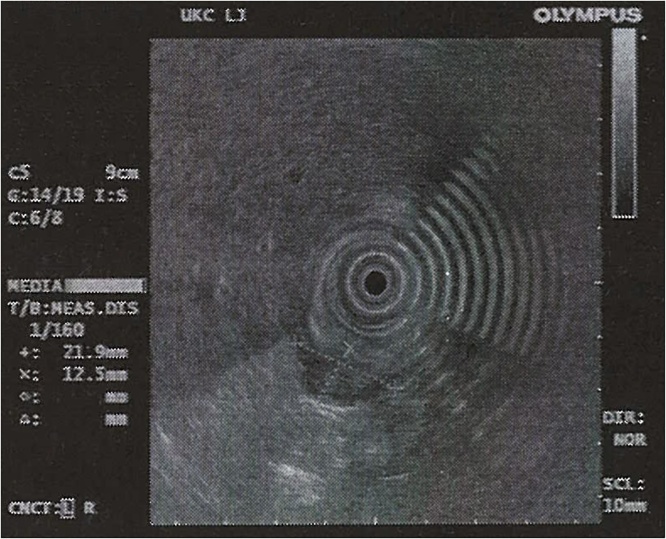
Endoscopic ultrasonography image of the gastric antral submucosal lesion, which ultimately proved to be a gastric ectopic pancreas. The lesion was growing from the fourth, muscular, layer of the stomach, measured 22 × 13 mm and displayed heterogenous echogenicity.
